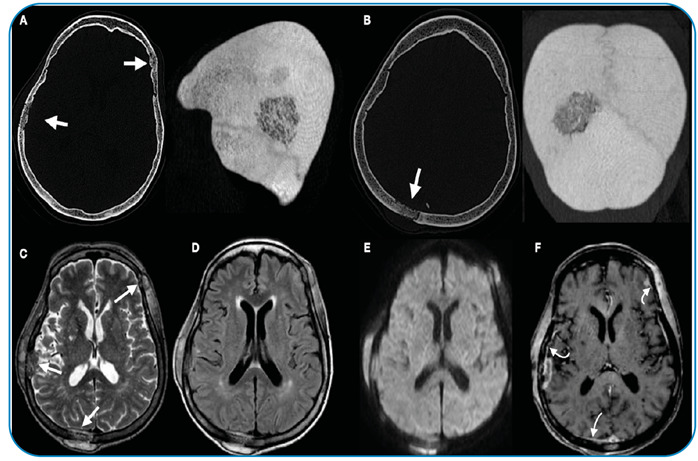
CT of the brain showing the permeative osteolytic pattern involving the left frontal and right temporal bones (A) and parietal bones (B). The permeative osteolytic pattern of the lesions is better demonstrated with MIP reconstruction. MRI of the brain showed lesions with a solid component and low signal on T2 WI (C) and on FLAIR WI (D), restricted diffusion on DWI (E), and heterogeneous enhancement on T1-WI after gadolinium in addition to pachymeningeal enhancement adjacent to the lesions (white curved arrow in F).
